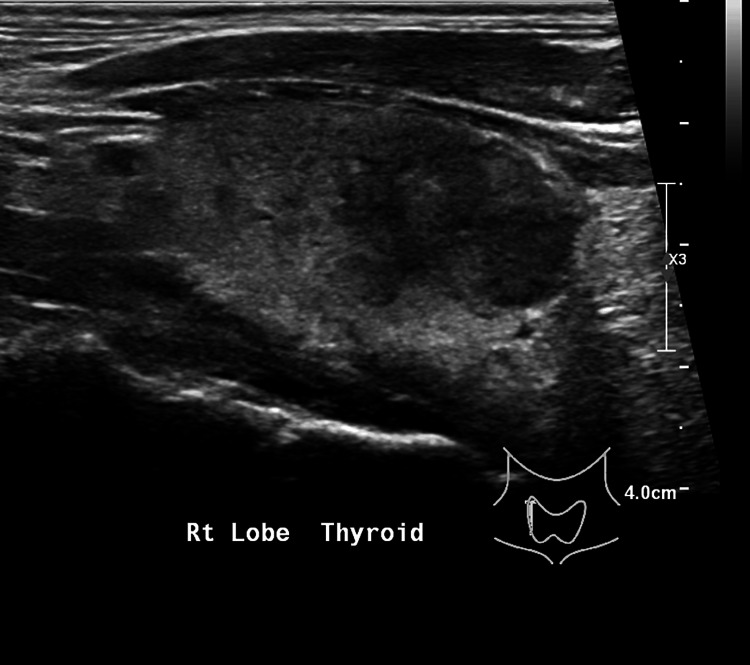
Similar features were seen on thyroid US of the right lobe. US: ultrasonography.
